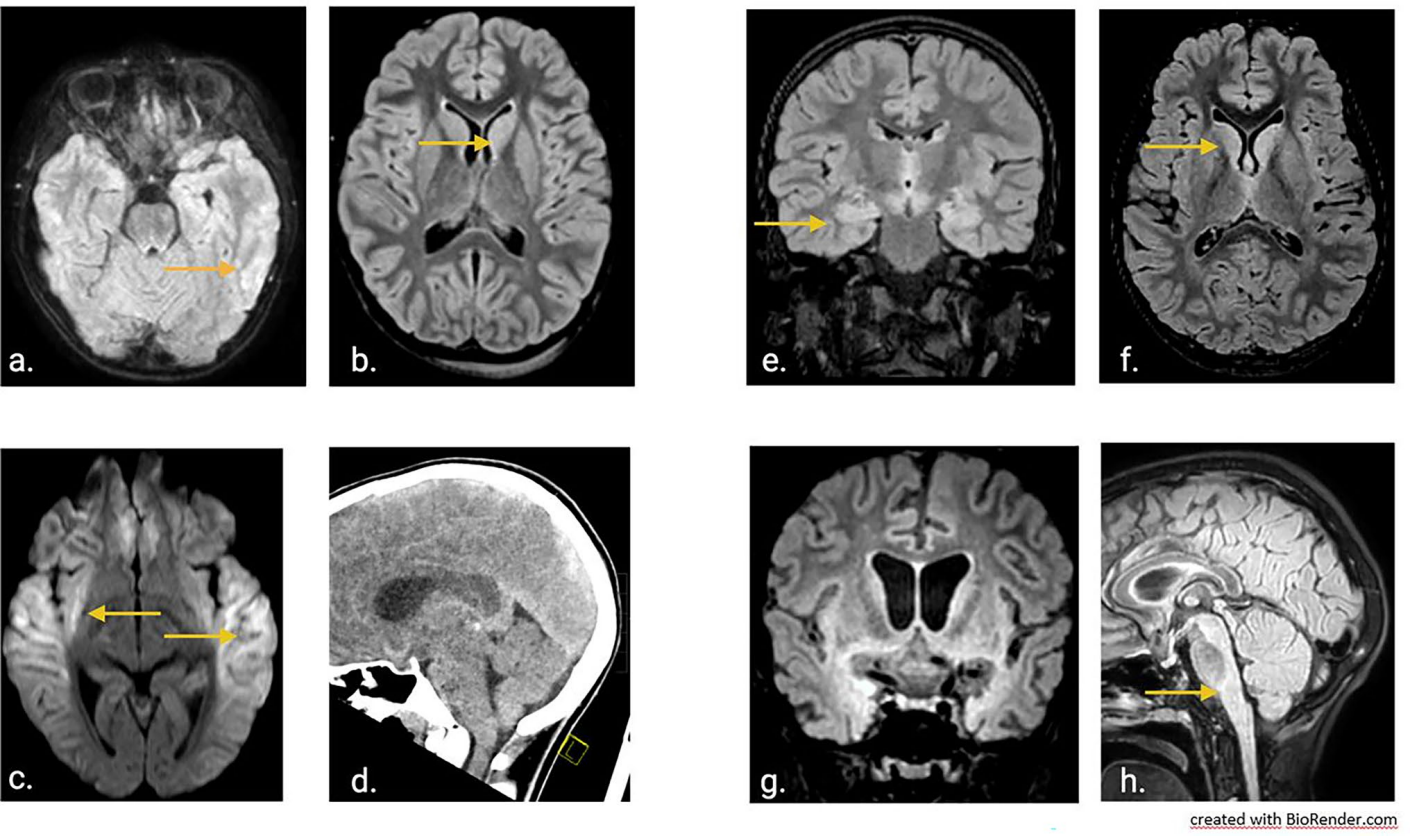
Brain magnetic resonance imaging of the two pediatric cases over time. Initial scans of Case 1 on day 8 after symptom onset showing signal hyperintensity in the parietal brain regions (fluid-attenuated inversion recovery (FLAIR) sequence) (a) and in the basal ganglia on the left on day 12 (FLAIR sequence) (b). Later scans of the same case on day 15 demonstrating signs of cytotoxic edema (diffusion weighted imaging) (c), and last computed tomography scan on day 29 with diffuse edema (d). Initial scans of Case 2 on day 12 of disease showing signal alterations in temporal brain regions (FLAIR sequence) (e) and in the basal ganglia (FLAIR sequence) (f). Late scans on day 51 demonstrating brain atrophy and signal hyperintensity in the basal ganglia and temporomesial (FLAIR sequence) (g), as well as in the brain stem (FLAIR sequence) (h)
